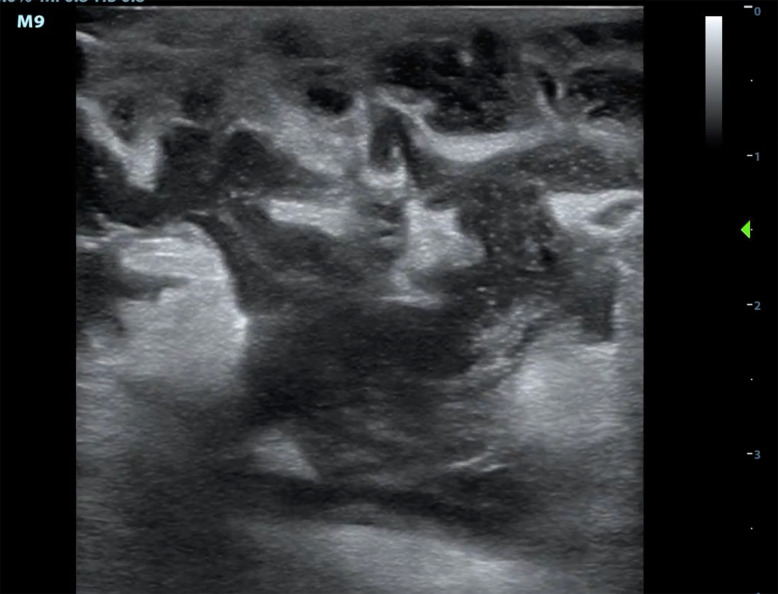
Breast point-of-care ultrasound examination showing dilated lactating ducts with surrounding abscess that required surgical consultation and drainage.
